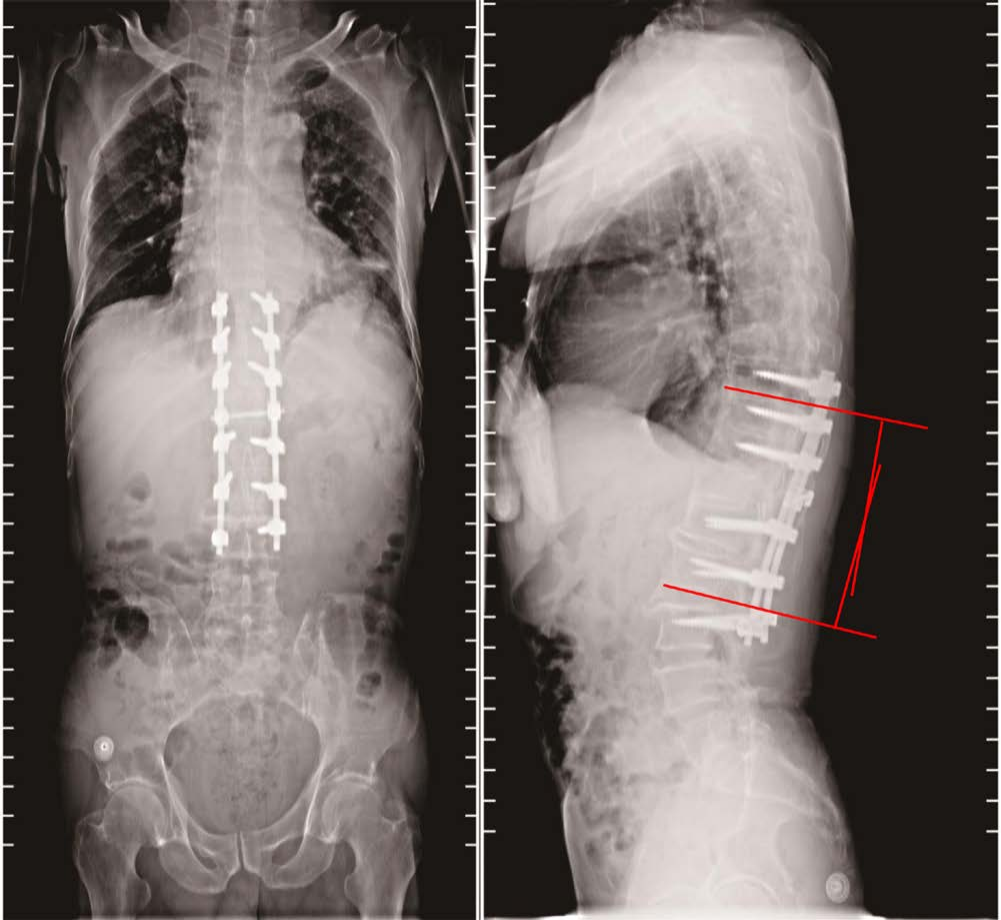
X-ray images of spine after surgery. The post-operative kyphosis Cobb angle is 7.7 degrees.
